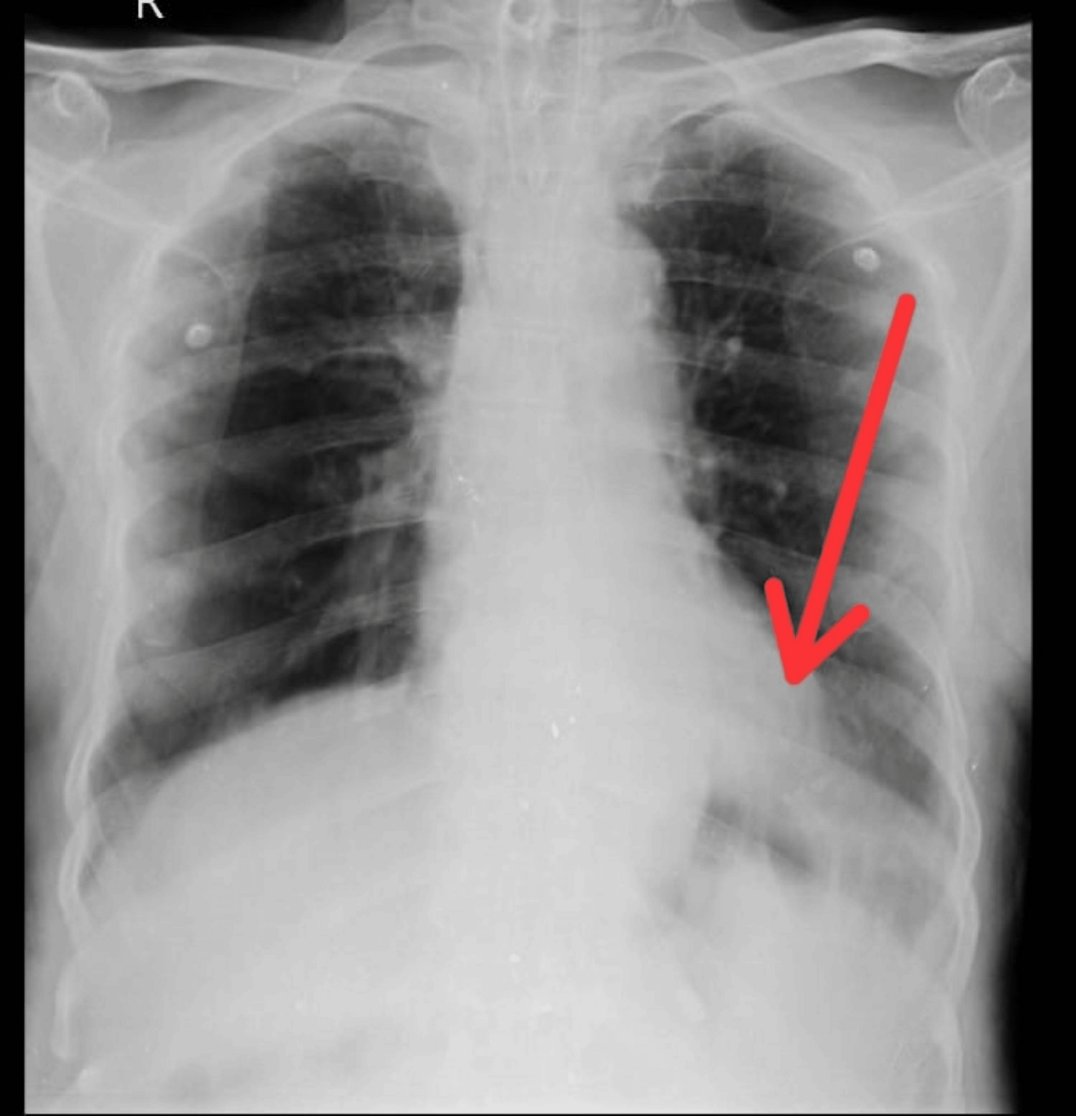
X-ray findings showing the bronchovascular markings present in the left lower zone and the right middle zone
